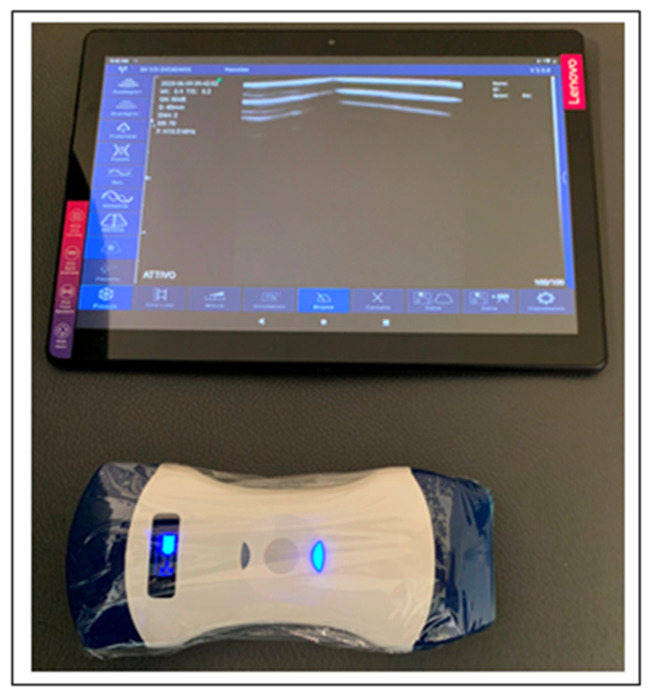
Wireless ultrasound equipment to avoid contamination. A wireless ultrasound probe with respectively a 4.5 mHz convex probe on the right side, and a 7.5 mHz linear probe on the left one. The transducer, wrapped with a single use plastic cover to avoid contamination, is wi-fi connected with the tablet which in turn, is encircled by a plastic film.
